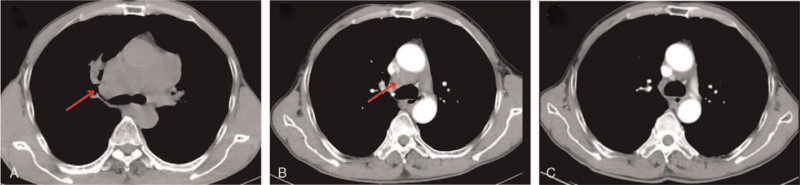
Chest CT of the patient after immunotherapy combined with chemotherapy/antiangiogenesis therapy. (A) Chest CT after camrelizumab combined with chemotherapy (June 19, 2019) showed the masses were larger than before. (B) The tumors significantly shrank after 4 cycles of camrelizumab combined with anlotinib (September 5, 2019). (C) The efficacy evaluation was CR (February 9, 2021). CR = complete remission, CT = computed tomography.
